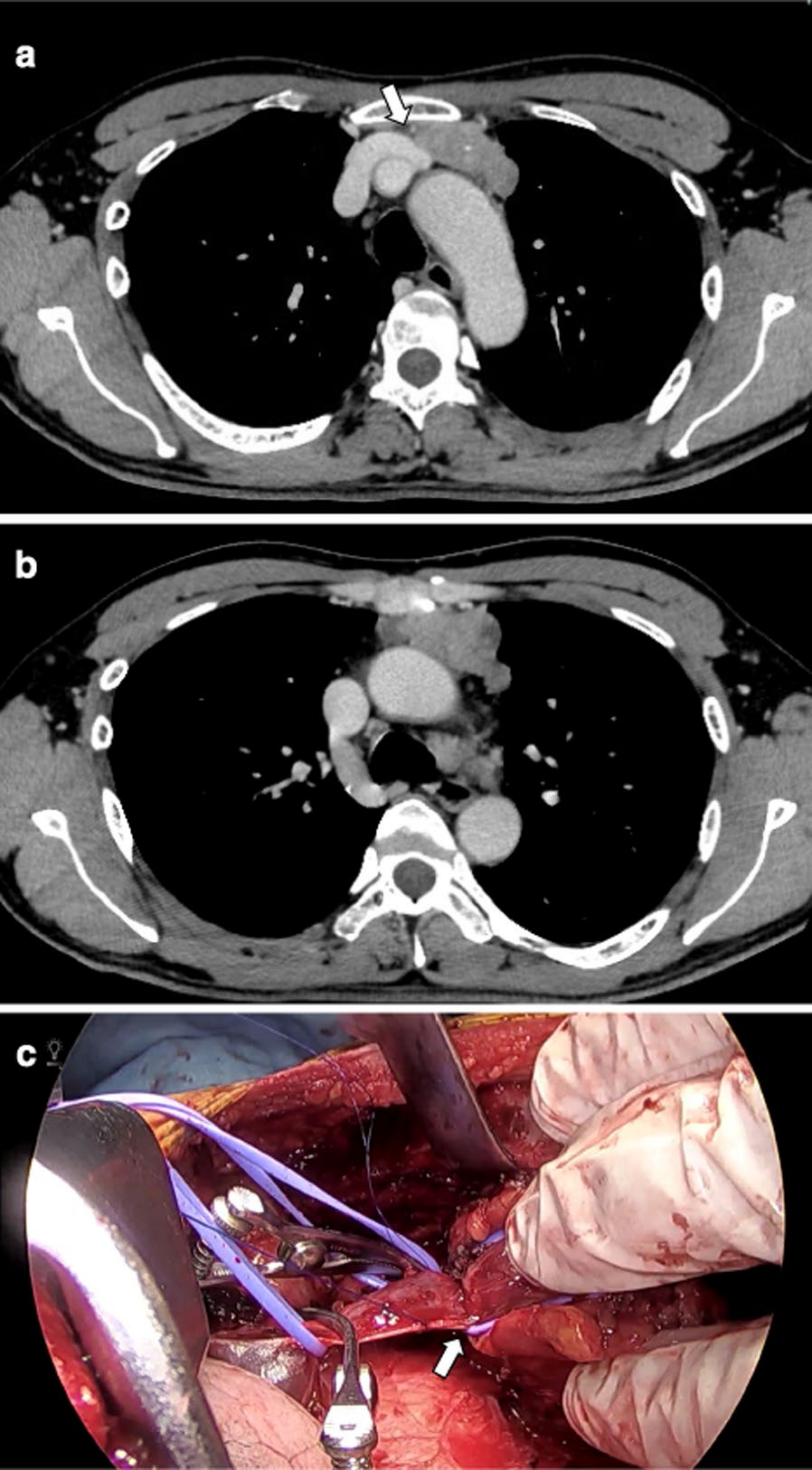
Preoperative chest computed tomography (CT) findings showed a 60 × 30 × 55 mm anterior mediastinal tumor with calcification. Contrast-enhanced CT showed mild encasement of left brachiocephalic vein (white arrow) and infiltration of the vein was suspected (a). The tumor contained the low-density areas and they might show necrosis or MTCs (b). Intraoperative findings showed strong adhesion of the left brachiocephalic vein (white arrow), and therefore partial resection of the vein was performed (c)
